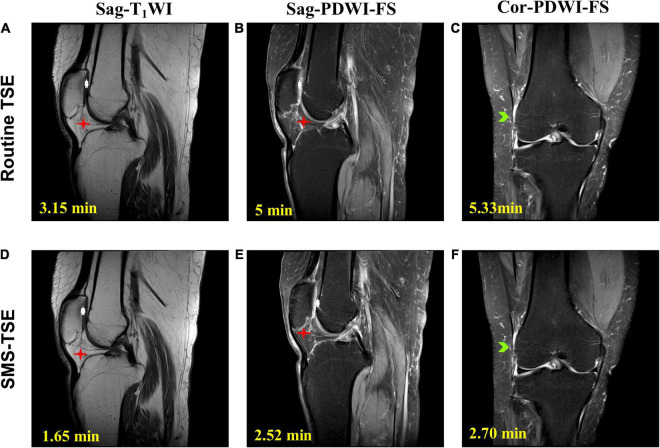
Comparison of the high-resolution turbo spin-echo (TSE) sequence and simultaneous multi-slice-TSE sequence in the knee joint imaging. On the magnetic resonance images, the degeneration of the knee joint is clearly depicted. All the images scored 5 in the image quality evaluation. For routine TSE, the SNR for Sag-T1WI (A), Sag-PDWI-FS (B) a d Cor-PDWI-FS (C) were 30.75, 72.86, and 78.67; and the CNR for each was 88.89, 44.39, and 43.18. For the SMS-TSE, the SNR for Sag-T1WI (D), Sag-PDWI-FS (E) a d Cor-PDWI-FS (F) were 29.22, 73.47, and 68.61; and the CNR for each was 77.11, 45.68, and 41.35.
